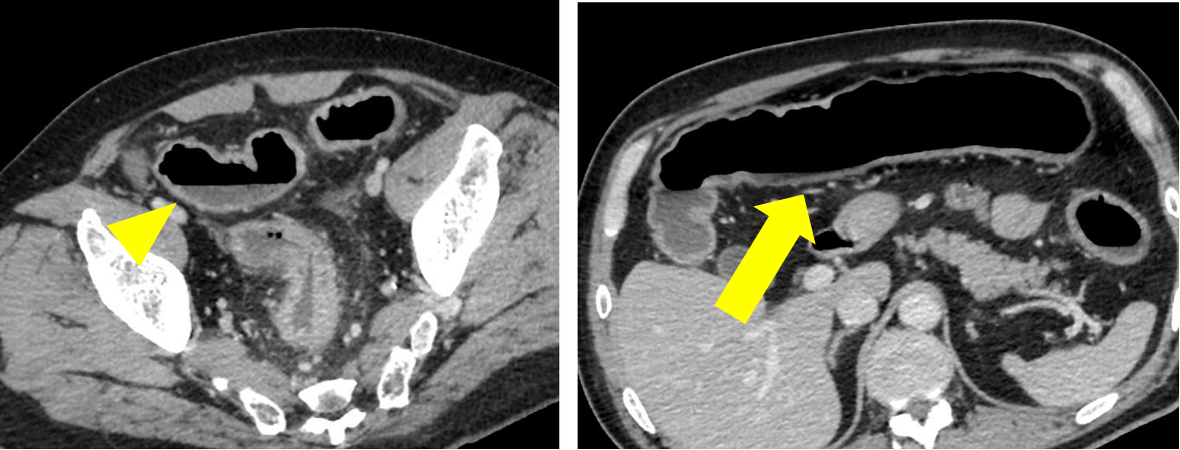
Second abdominal CT scans. CT revealing extensive edema and dilated lumen without haustra in the entire colon; transverse colon (arrow) and cecum (arrowhead)
